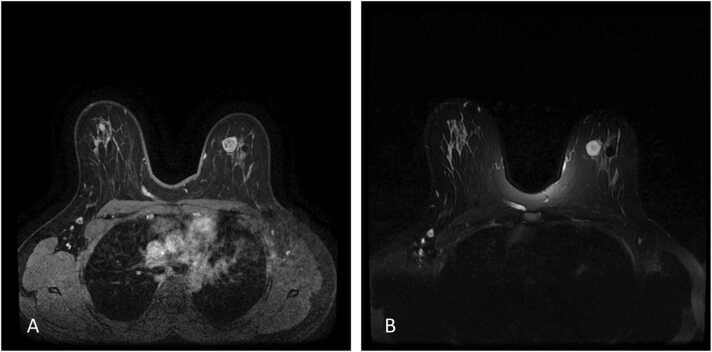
Axial T2-weighted (A) and axial post contrast image (B) demonstrate a circumscribed round mass with high signal intensity on T2-weighted and heterogenous enhancement with surgical excision pathology result of fibroadenoma.
